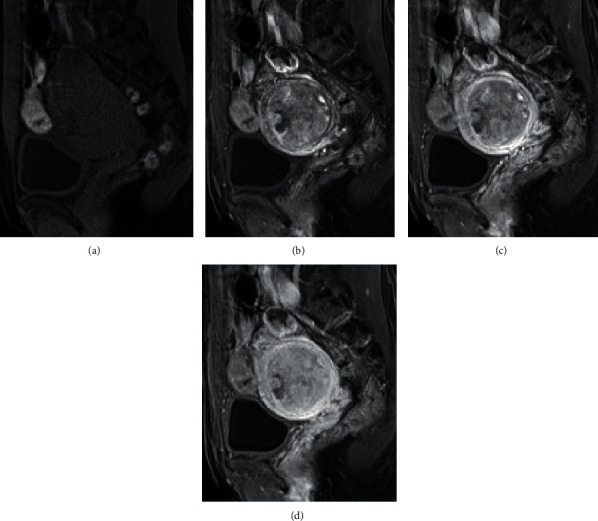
Dynamic contrast-enhanced T1-WI pelvic MRI: (a) precontrast; (b) postcontrast 20 s; (c) postcontrast 60 s; (d) postcontrast 180 s. In dynamic contrast-enhanced T1-WI, the small nodules are enhanced rapidly and stronger than the normal myometrium in the early phase and decrease gradually in the later phase to the level similar to that of the normal myometrium. In contrast, the larger mass is enhanced gradually and heterogeneously, but it is weaker than the normal myometrium.
